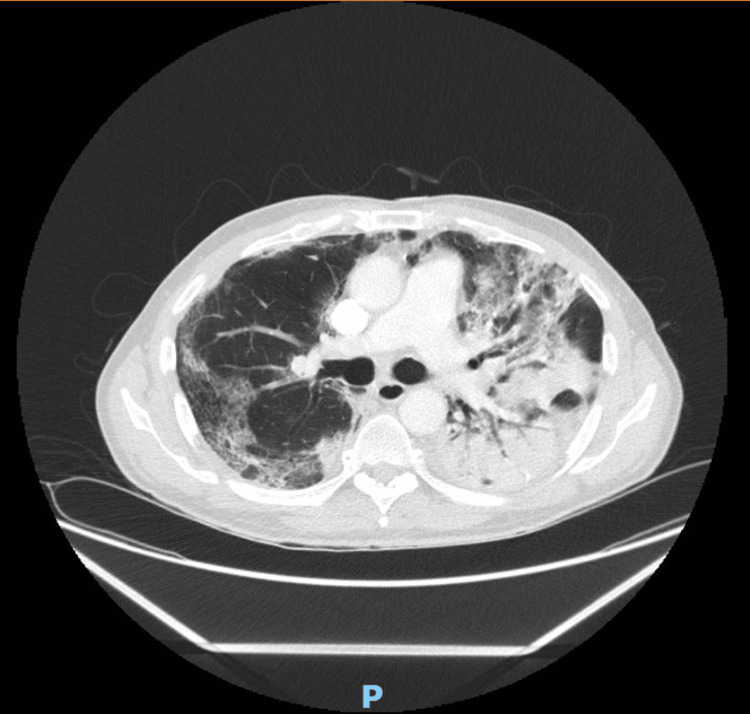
CT thorax previous admission four days before discharge
